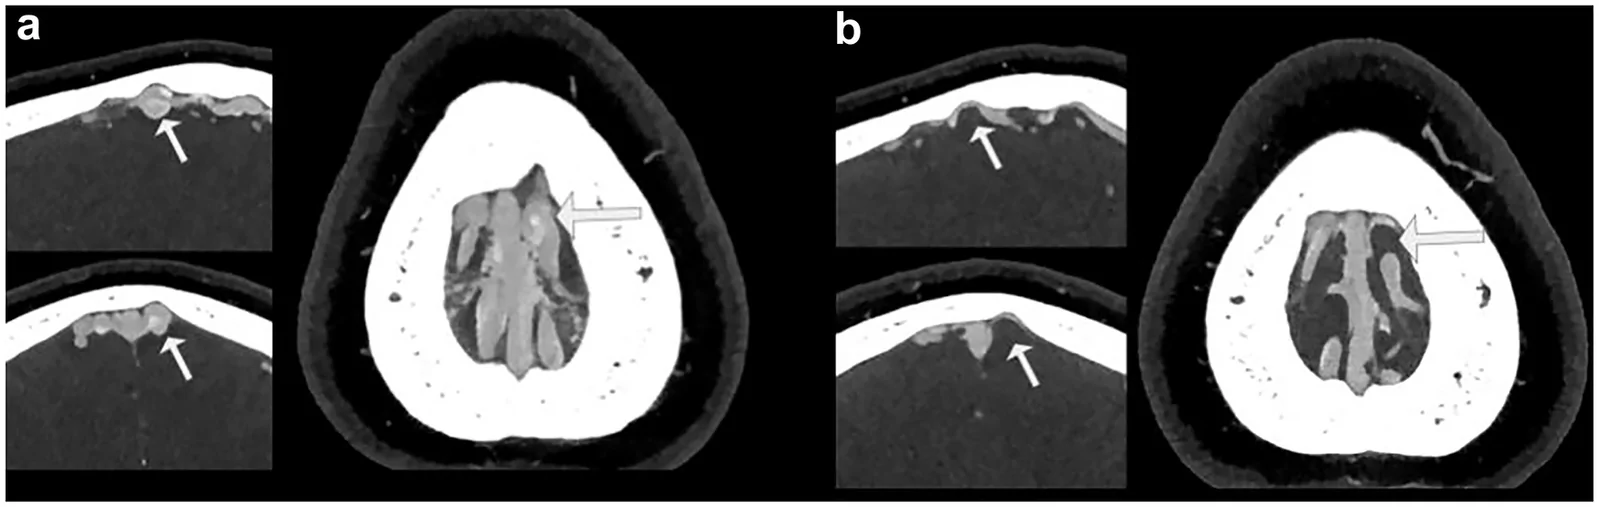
Venous changes in intracranial hypotension. a CT venography after dural puncture shows diffuse venous engorgement and absent arachnoid granulations (arrows). b Follow-up demonstrates normalized venous caliber with visible arachnoid granulations (arrows), indicating normalized intracranial pressure
